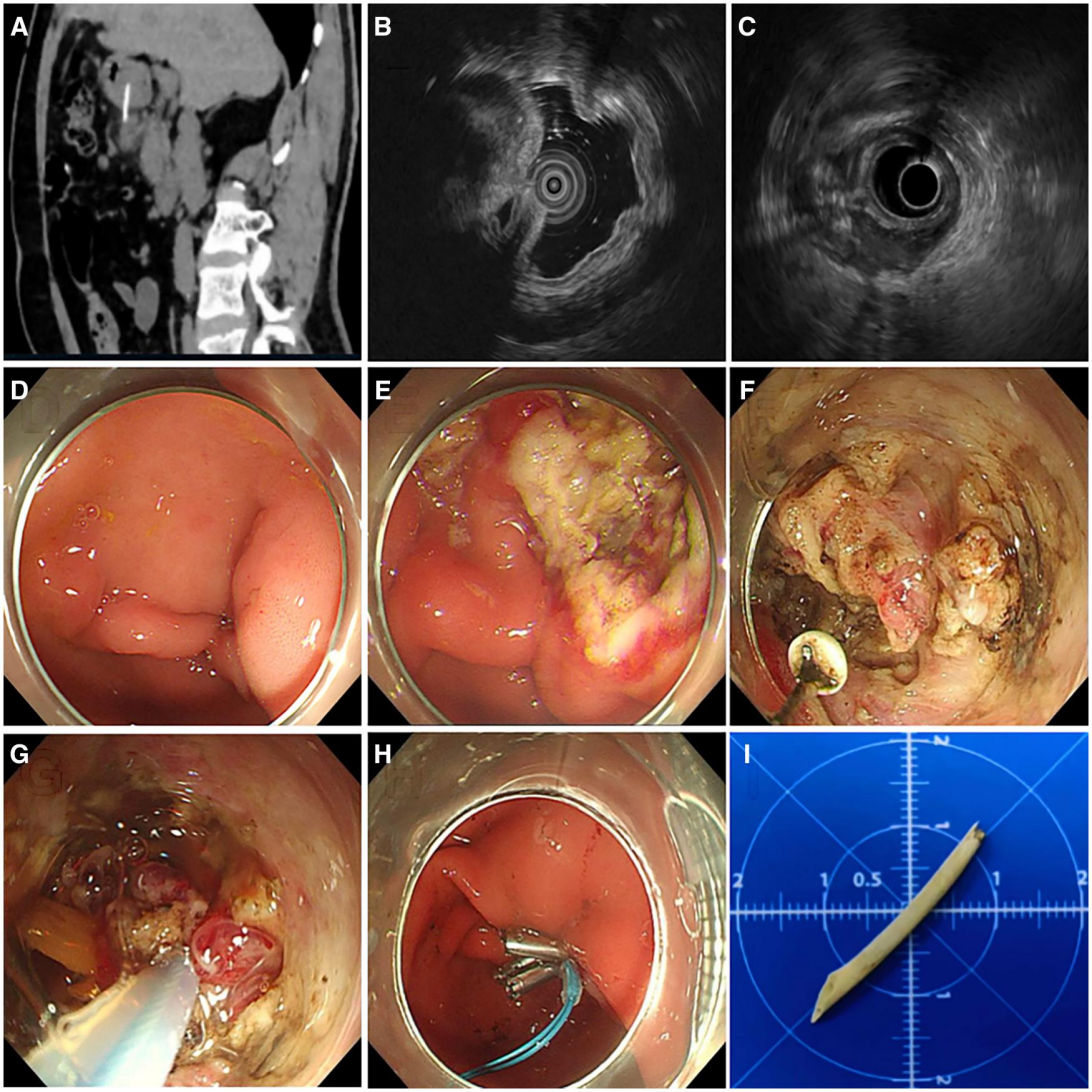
Endoscopic removal of gastric antral-embedded foreign body (FB) in one patient with a 1-month history of abdominal distension and pain. (A–C) Localization of the FB: pre-operative visualization of the FB under computed tomography (A) and intraoperative 20 MHz (B) and 6 MHz (C) endoscopic ultrasonography. (D) Mass with umbilication under endoscopy. (E) Submucosal injection and overlying mucosal flap removal. (F) Granulation tissue dissection, myotomy, and FB exposure. (G) FB extraction. (H) Hemostasis and closure of the gastric wall wound. (I) The FB was a 3-cm-long linear fish bone.
